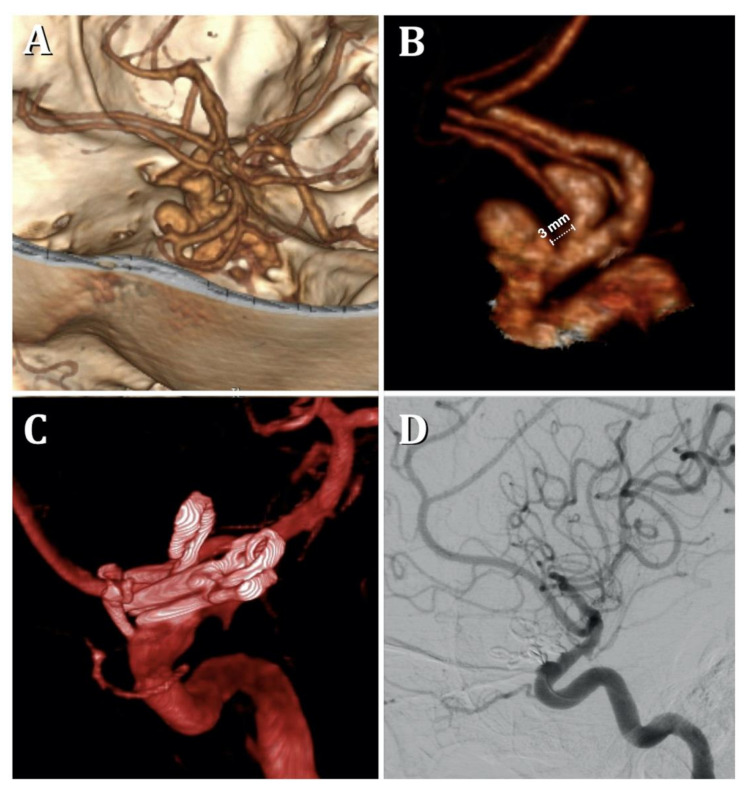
(A,B) Preoperative 3D CT angiography showing the left tandem paraclinoid internal carotid artery aneurysms. Postoperative 3D (C) and 2D (D) digital subtraction angiography in lateral projection.
